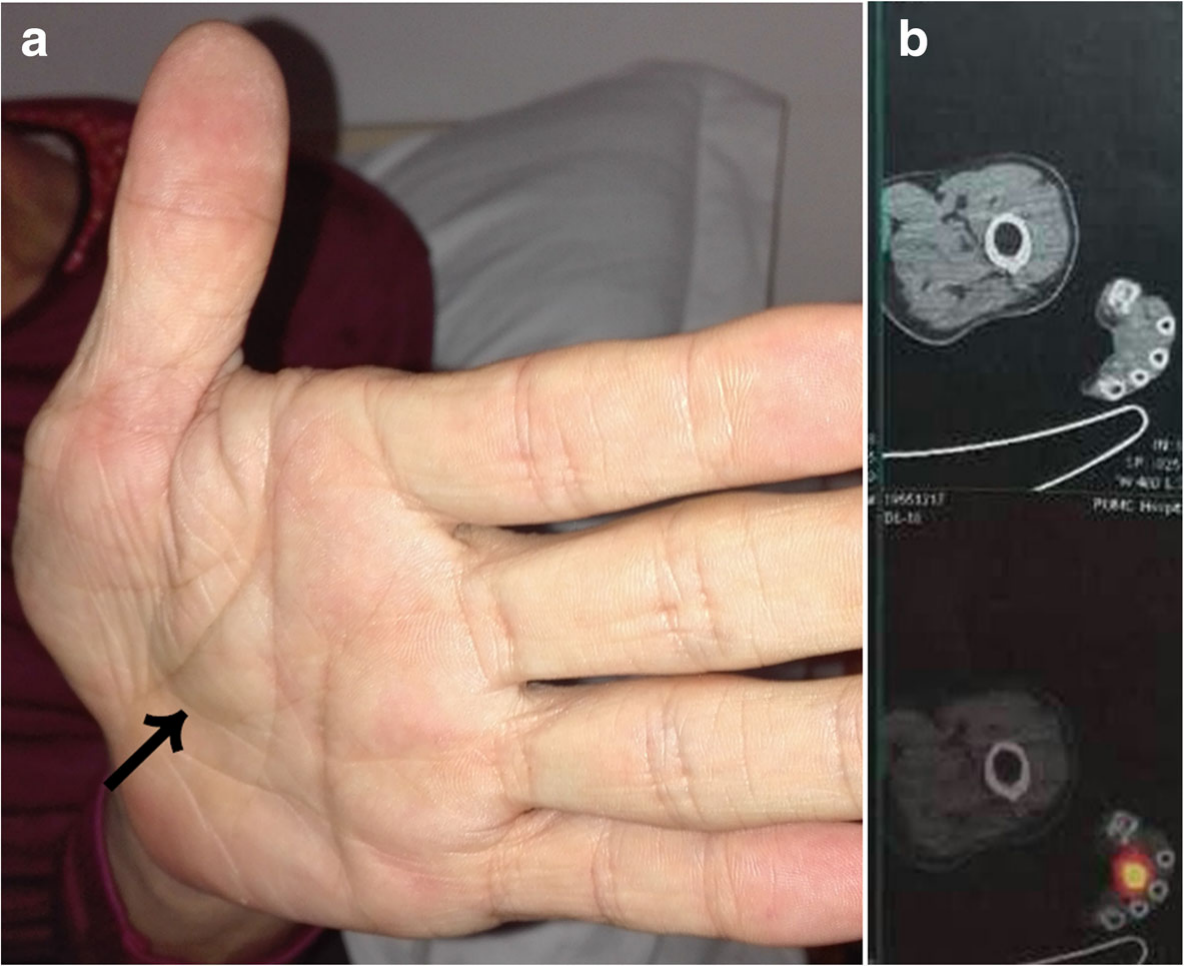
Patient 9. (a) A tumor was visible and palpable in the soft tissue of the left palm (arrow). (b) ^18^F–FDG PET/CT showed high ^18^F–FDG uptake in the tumor
